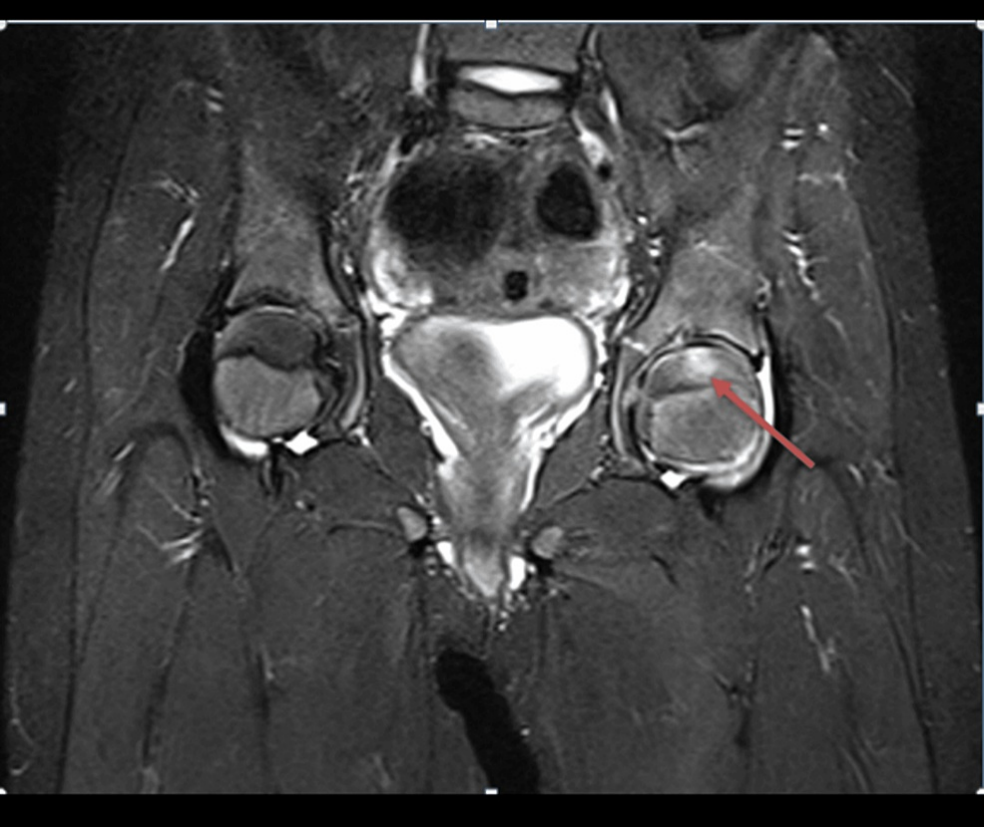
Coronal hip MRI (T2-weighted image with fat saturation) showing a band-like rectangular focal abnormally high signal on the left femoral head (red arrow).
